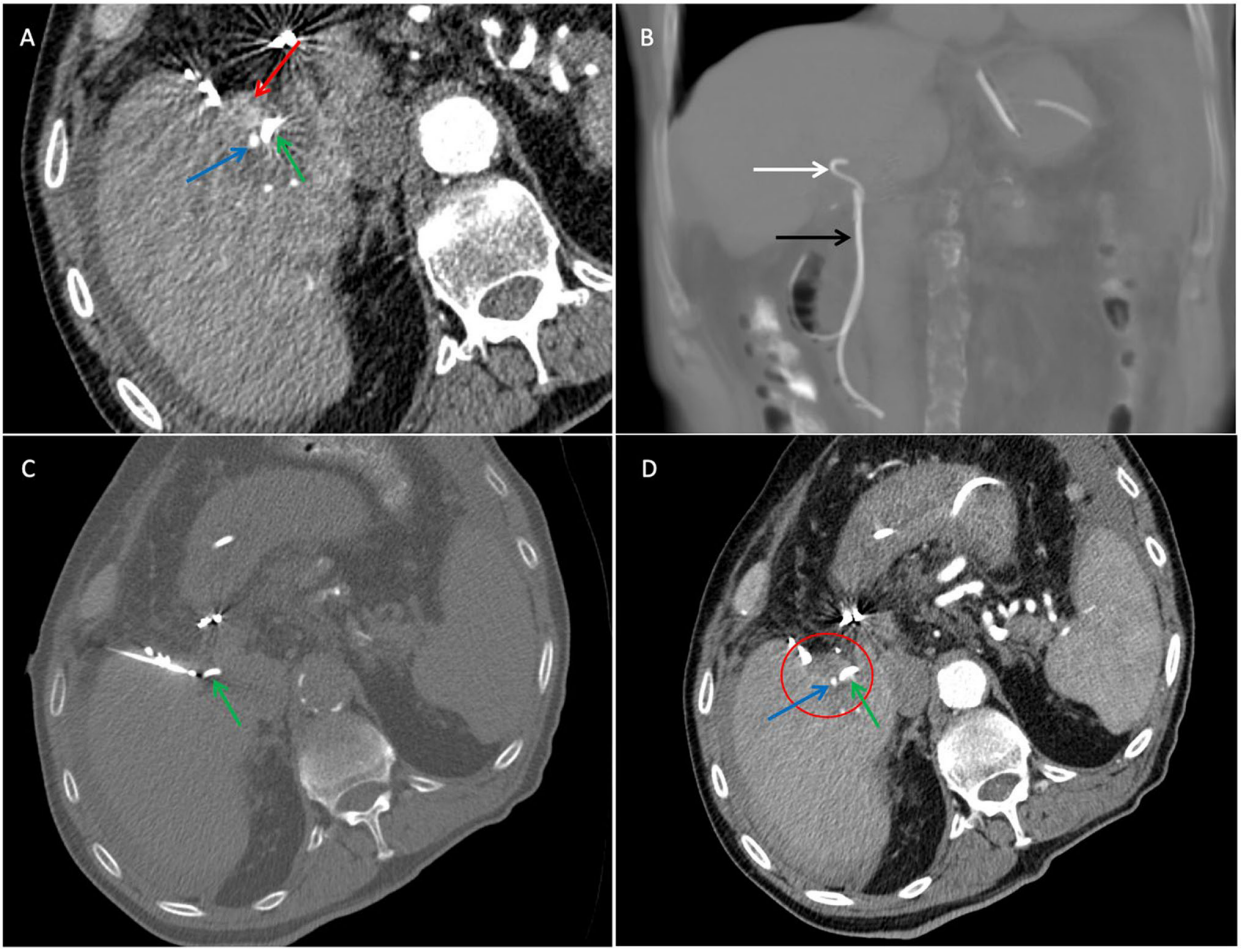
CT-guided cryoablation of patient 1 with HCC next to the right hepatic duct. A: Pre-ablation planning CT (axial in arterial phase) shows a 15-mm HCC with early contrast enhancement next to the right hepatic duct and hepatic artery. The minimum distance to the right hepatic duct was 0 mm. B: Pre-ablation planning CT (coronal without contrast medium) shows the nasobiliary tube and biliary stent, which was placed 34 mm into the right hepatic duct before cryoablation. C: Position of the cryoprobe just before ablation (CT axial without contrast medium). D: Post-ablation CT (axial in arterial phase) shows complete necrosis of the HCC with inflammatory rim enhancement.*red arrow: tumor; green arrow: bile duct; blue arrow: hepatic artery; black arrow: nasobiliary tube; white arrow: biliary stent. *HCC: Hepatocellular carcinoma
